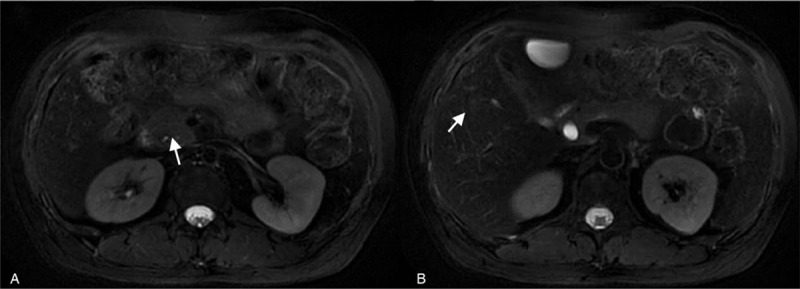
A: After 2 cycles of chemotherapy, MRI showed that the lesions in the uncinate process of the head of the pancreas were significantly reduced (white arrow); B: The focus of the liver has also become blurred. (white arrow).
